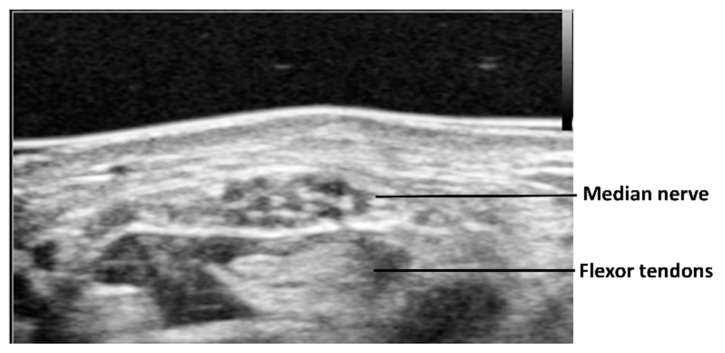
A typical ultrasound image of median nerve at proximal carpal tunnel.
